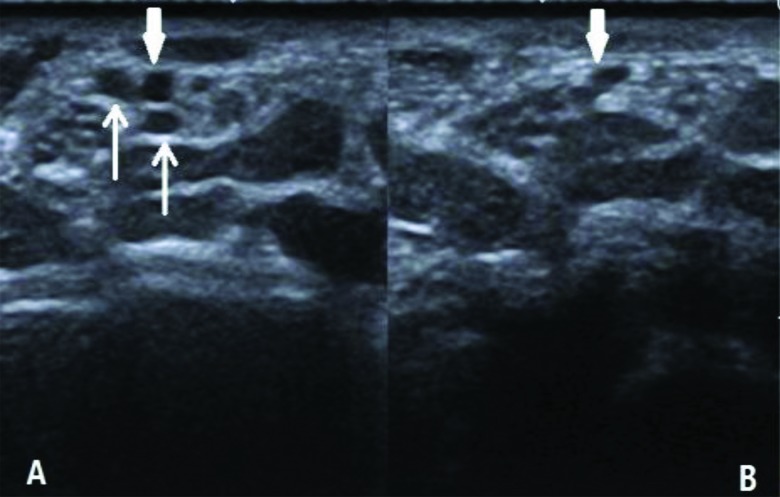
Persistent median veins are often accompanied with PMA (in Figure 4A, thin arrow). The veins become deformed and invisible (Figure 4B) when additional force is applied to the surface of the wrist during the ultrasonography examination. A persistent median artery is difficult to deform (thick arrow).
